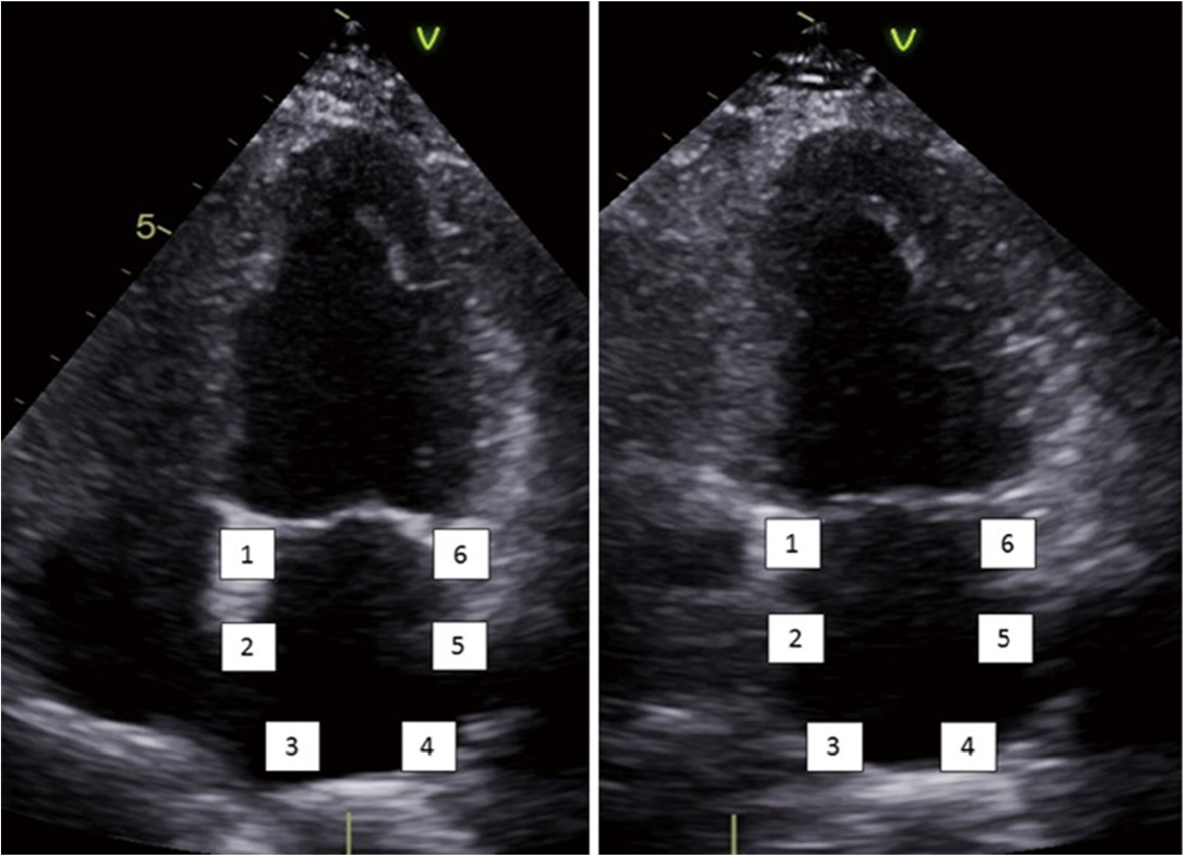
Apical four-chamber and two-chamber views of the six left atrial segments
